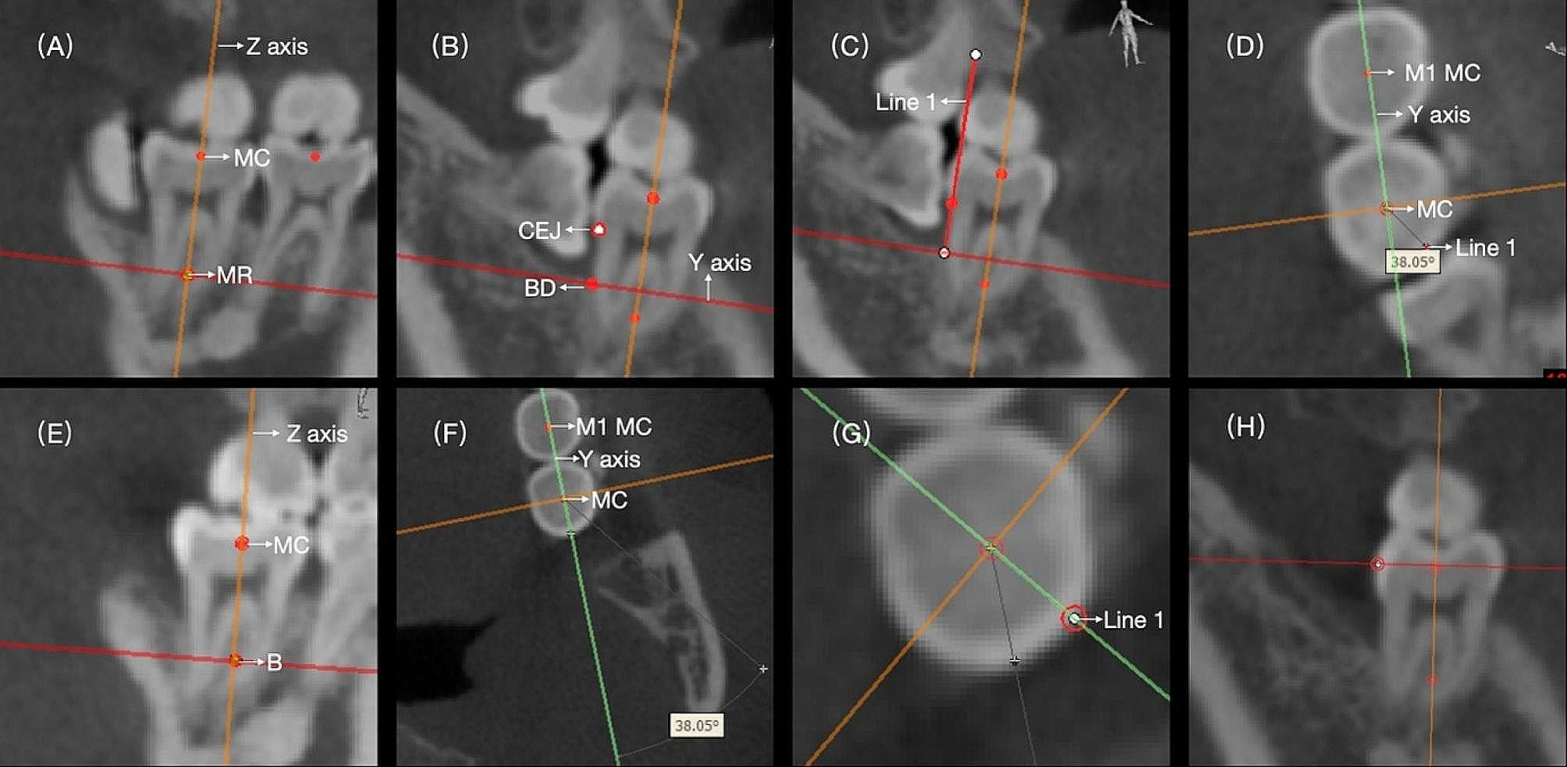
To establish a consistent measurement plane: (A) Verify that the Z-axis intersects the central axis of M2 on the CBCT before baseline. Define the central axis of M2 by a line passing through the midpoint (MC) of the mesiodistal crown width and a point one-third of the distance from the apex of the mesial and distal roots. (B) Locate the base of the defect (BD) and place it on the plane intersecting BD and the central axis (Z-axis). This plane is the measurement plane. (C) In the measurement plane, create a line (Line 1). (D) Return to the transverse plane at point MC. Line 1 should correspond to a point within this plane. Rotate the Y-axis across the midpoint of the mesiodistal crown width of M1 and measure the angle formed by Line 1, point MC, and the Y-axis. (E) Locate the Z-axis on the central axis at the 1-year post-operative CBCT. (F) Create an angle identical to the one formed by Line 1, point MC, and the Y-axis. (G) This final step determines the measurement plane on the post-operative CBCT
